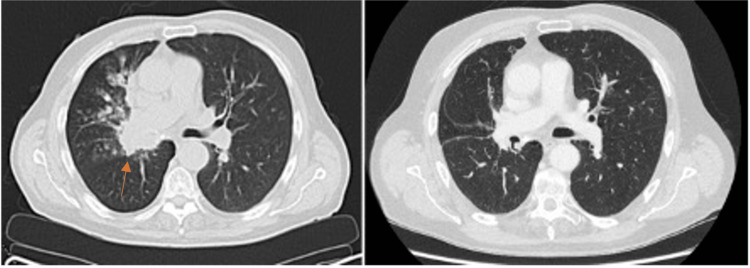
Initial CT scan demonstrating right perihilar lesion (left panel). CT scan post-chemo-radiotherapy demonstrating a reduction in tumor size (right panel).
